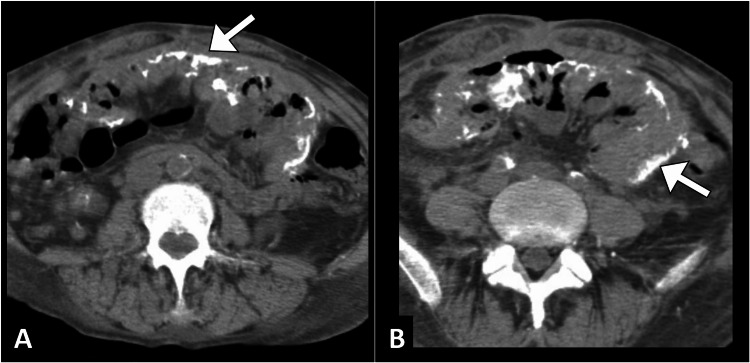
Axial CT images of the abdomen (A and B) demonstrating the encapsulation of small bowel loops by a calcified fibrous membrane (arrows), revealing the characteristic "cocoon-like" appearance indicative of sclerosing encapsulating peritonitis CT: computed tomography
